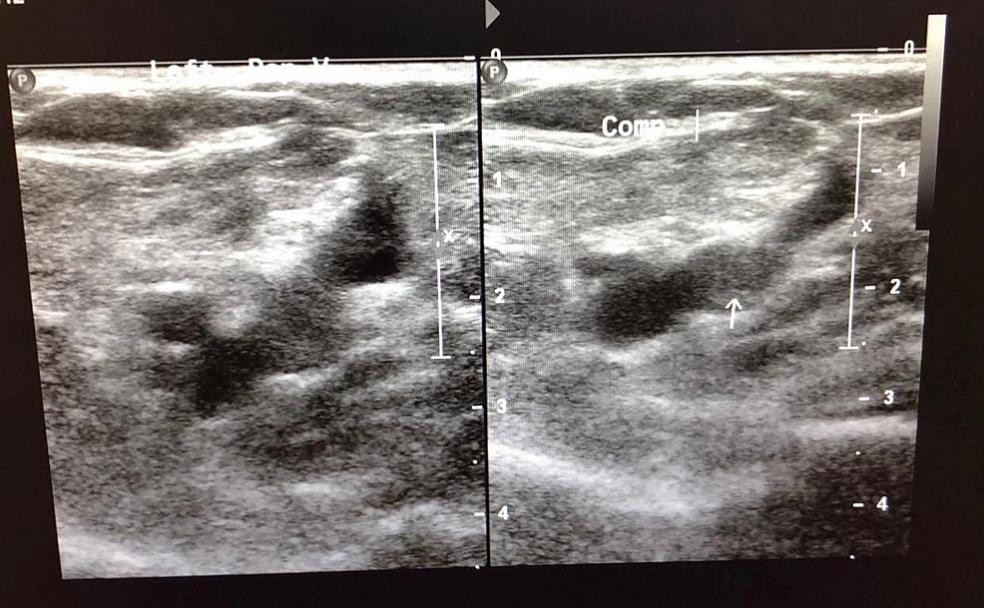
Doppler ultrasound scan image showing thrombus in left popliteal vein
